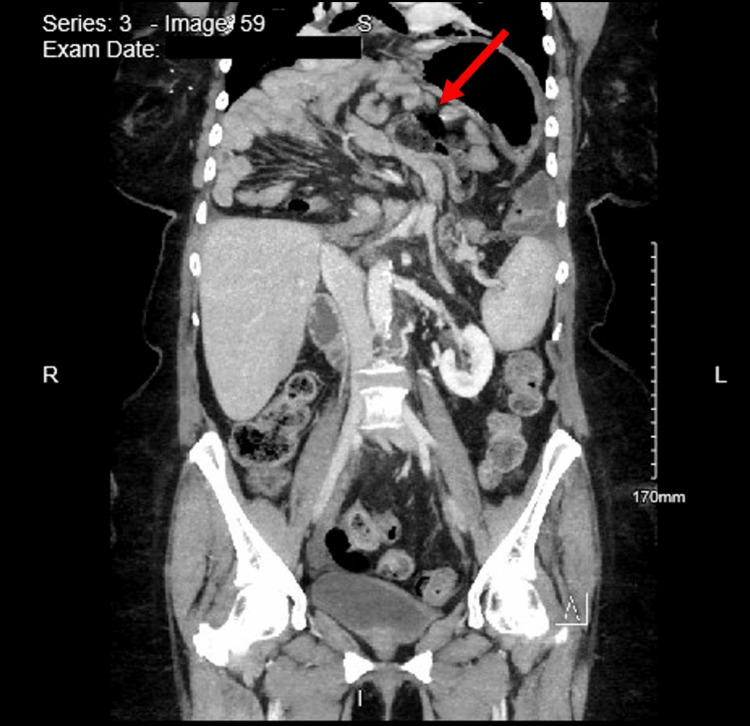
CT taken 4 months after the initial presentation The image showed a very large hiatal hernia containing the stomach, some small bowel loops, and a portion of the transverse colon. Some surrounding ascites were also observed CT: computed tomography
